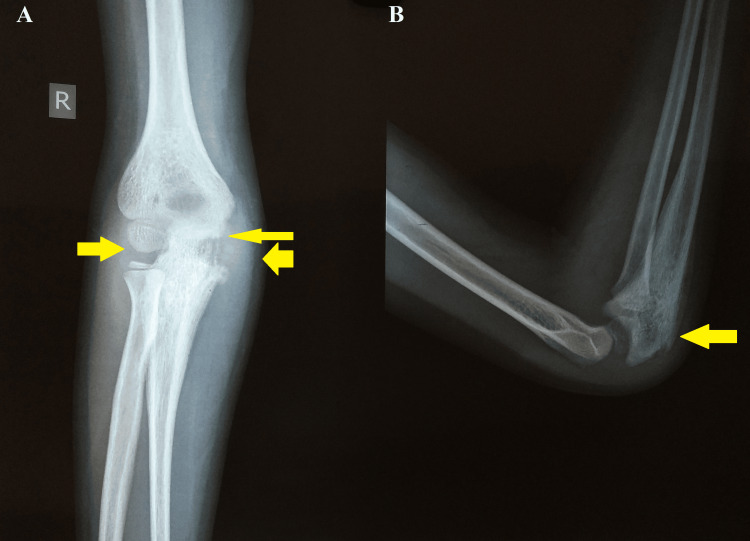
Anteroposterior and lateral radiographs of the right elbow joint radiographs showing erosion of the distal cortex of the humerus, olecranon process of the ulna, and radial bone head with swelling of the soft tissues around the right elbow joint A: Anteroposterior view (arrows showing erosion of the distal cortex of the humerus, olecranon process of the ulna, and radial bone head with swelling of the soft tissues around the right elbow joint). B: Lateral view
